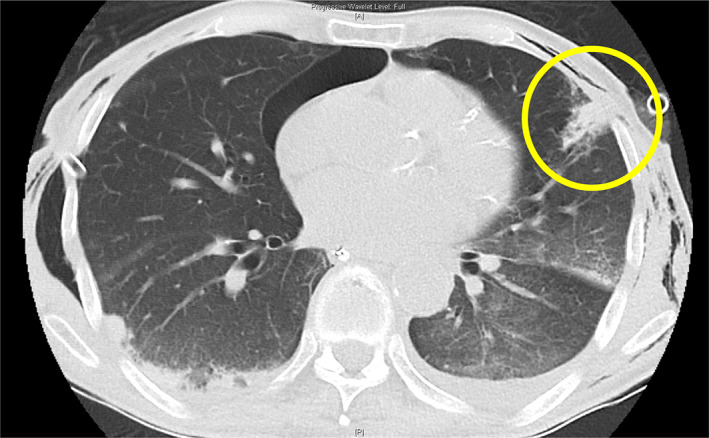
CT scan demonstrating left lung injury (circled) following Kelly clamp pleural puncture.
